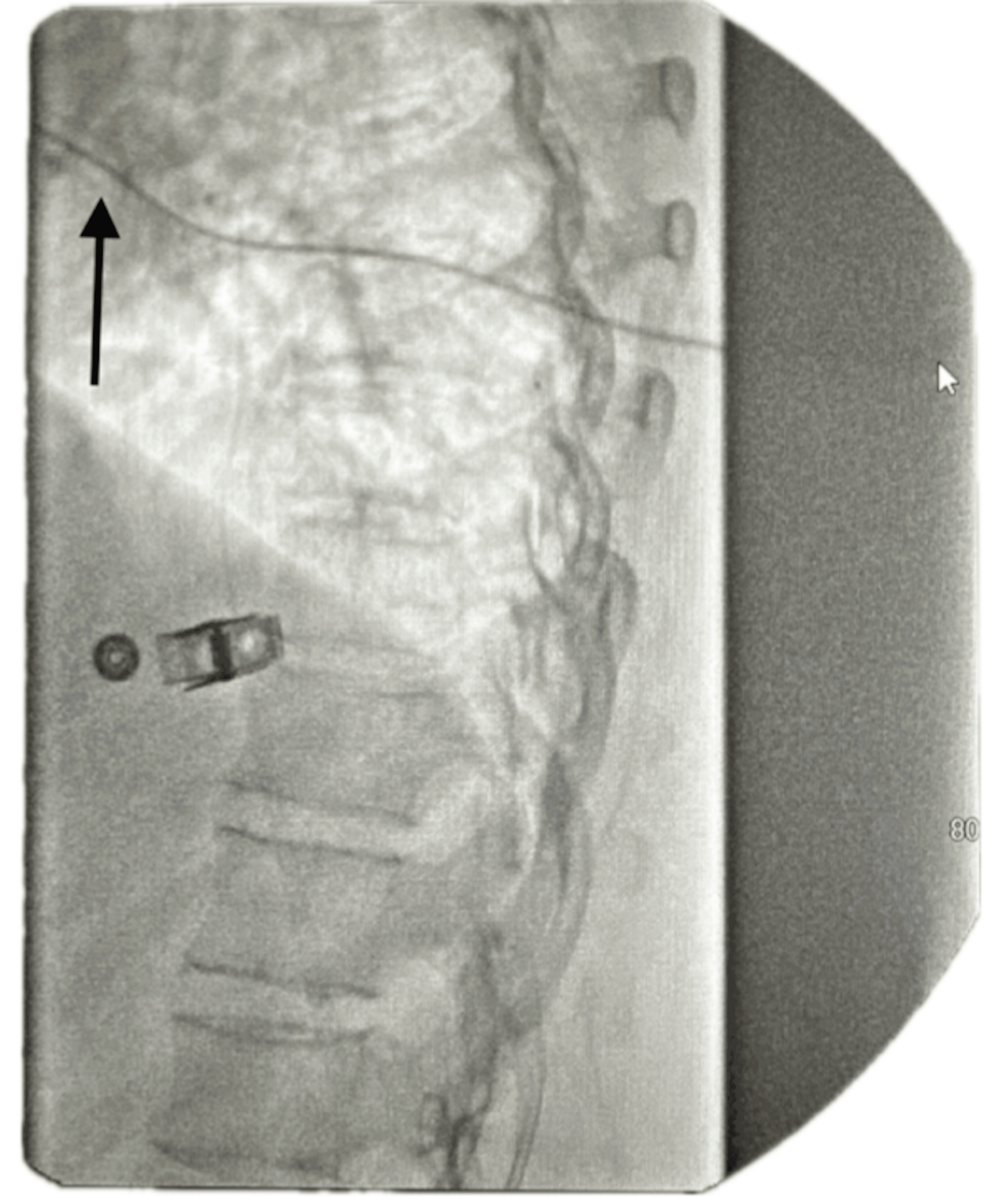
Lateral lumbar X-ray view demonstrating intrathecal catheter tip placement. The black arrow on the left highlights the path of the catheter extending toward the spinal canal.
